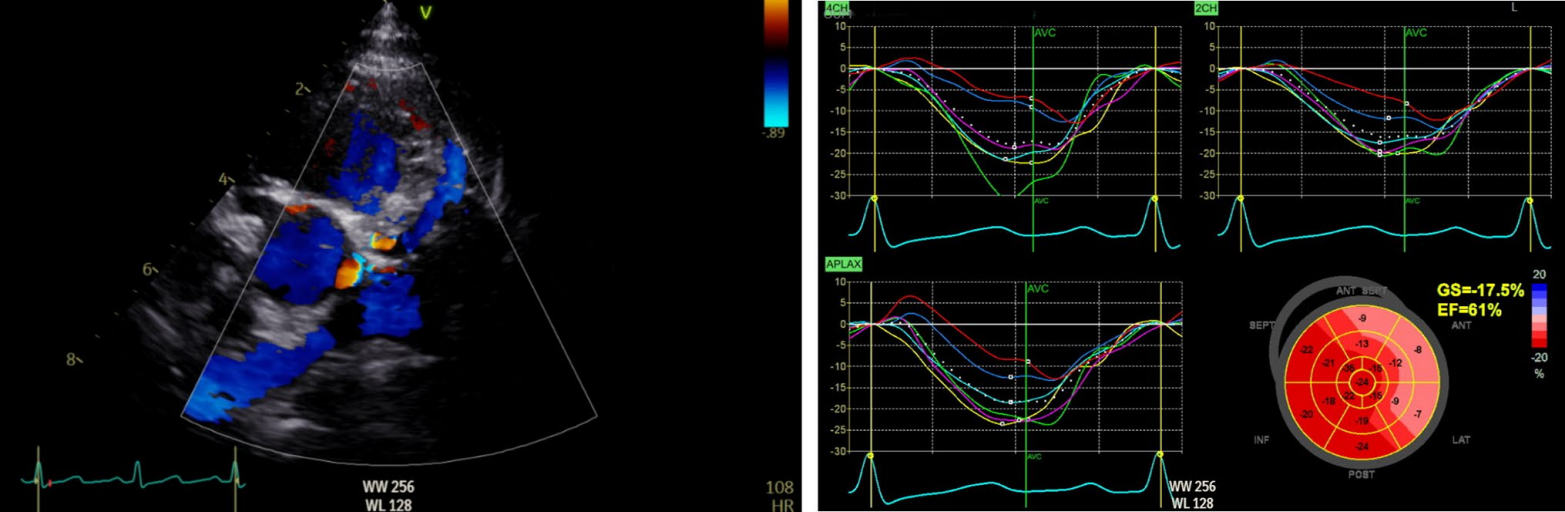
On the left panel, parasternal short axis with color doppler demonstrating a dilated right coronary artery (RCA) and the left coronary artery arising from the posterior side of the pulmonary trunk with retrograde flow. On the right panel, Global Longitudinal Strain subtle regional abnormalities in the anterolateral territories
